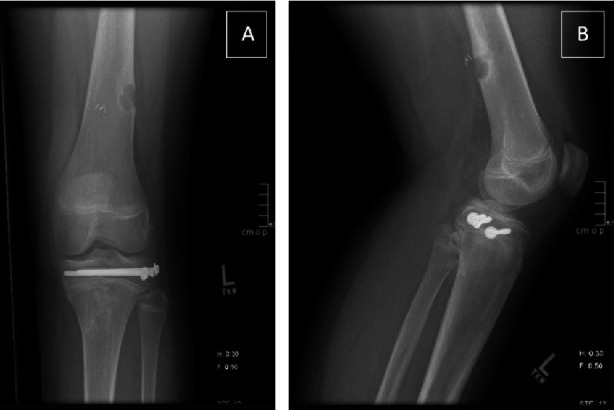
Postoperative knee radiograph demonstrating screw fixation with anatomic reduction and healing of displaced proximal tibial epiphyseal with metallic clips from vascular surgery. (a) Lateral; (b) anterior posterior.
